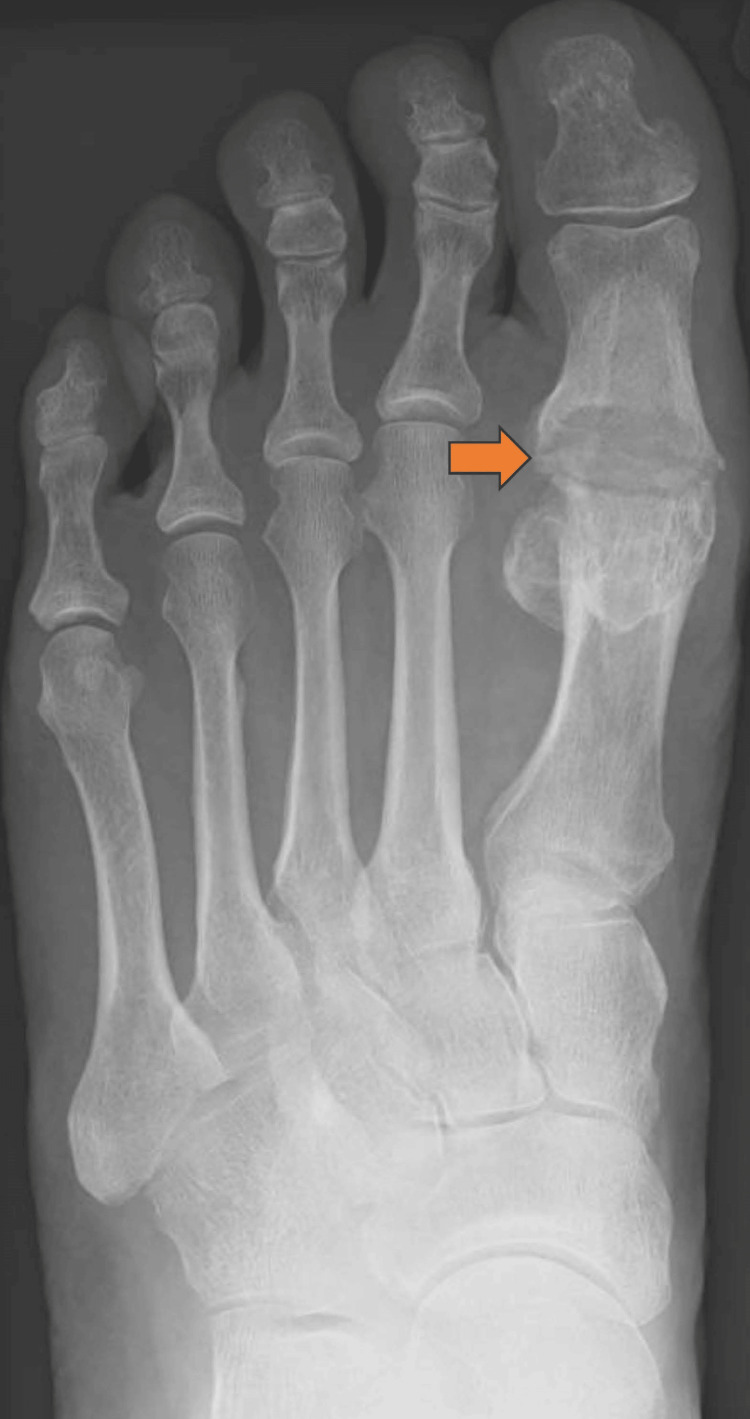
Post-operative Radiograph Showing Silastic First Metatarsophalangeal Joint Replacement
